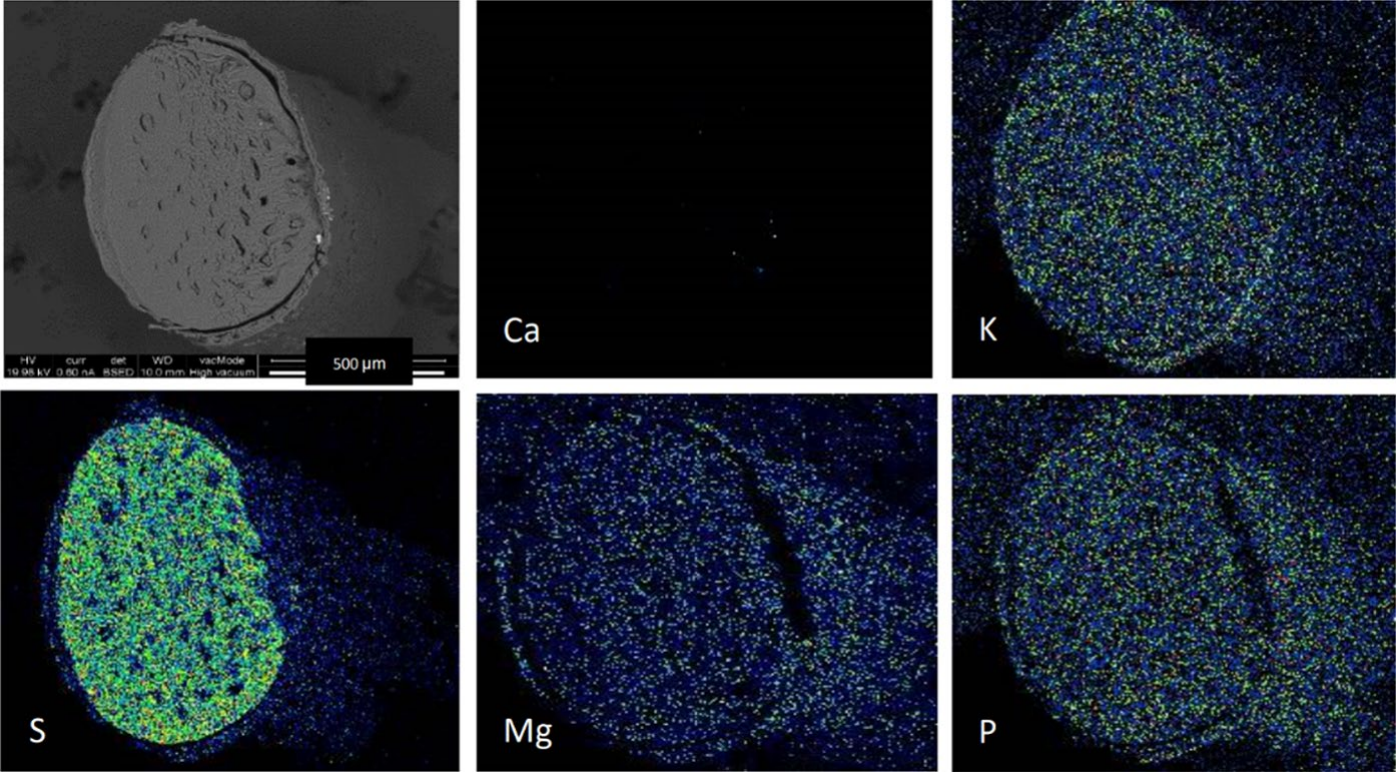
Energy-dispersive X-ray microanalysis (EDXA) elemental mapping of a cross-section of a wild female elephant tail hair. Black represents zero background through green, yellow and orange representing low to intermediate concentration to red or white, representing high concentration. The SEM image in the top left-hand corner shows the overall structure of the hair. Ca = calcium, K = potassium, S = sulphur, Mg = magnesium and P = phosphorus
